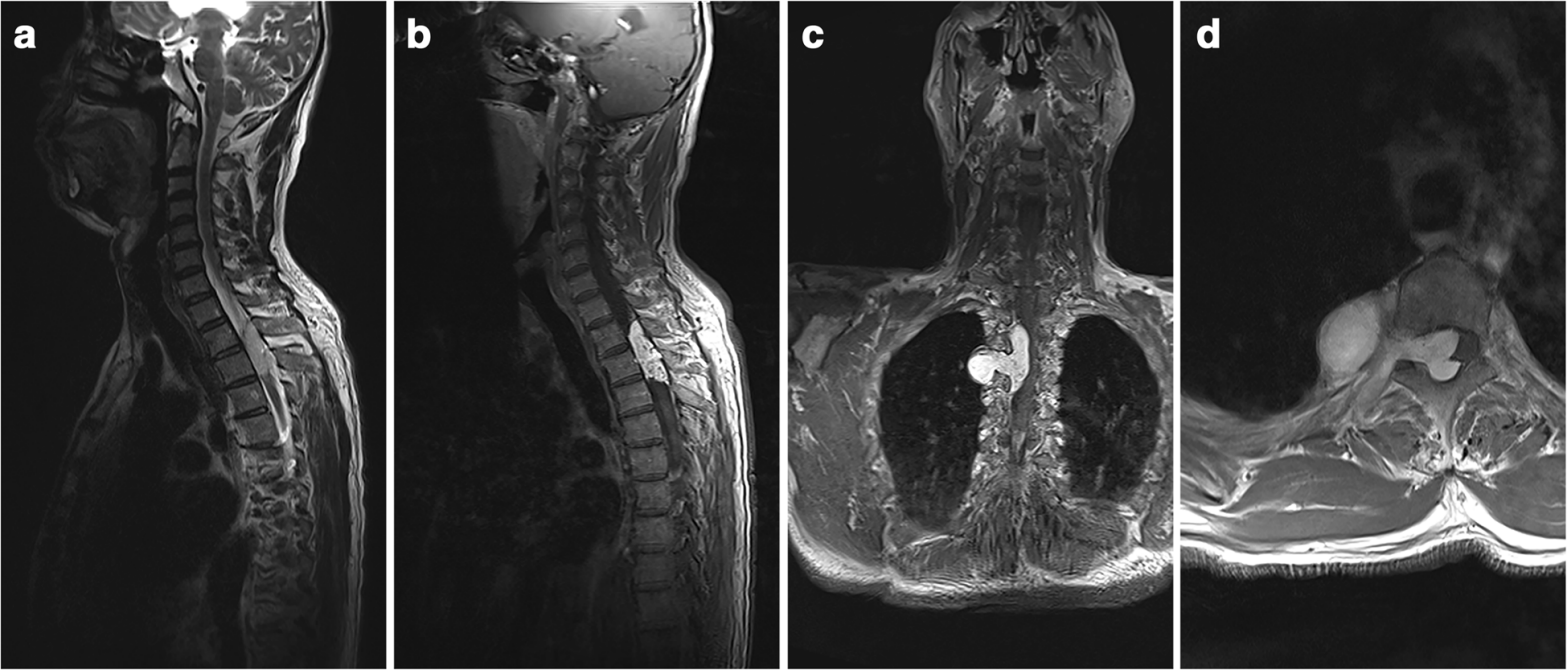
Preoperative sagittal T2-weighted magnetic resonance images (a) and contrast image (b, c and d) shows an epidural lesion from T2 to T3 level, extending through ipsilateral conjunction foramen and with intrathoracic extension (b, c and d). The epidural mass revealed isosignal intensity on T1-weight sequence and hypersignal intensity on T2-weighted sequence, with sagittal, axial and coronal view of homogeneous-enhanced after gadolinium injection. The lesions demonstrated isosignal intense in CT scan, without vertebral body erosion and enlargement of the intervertebral foramina
